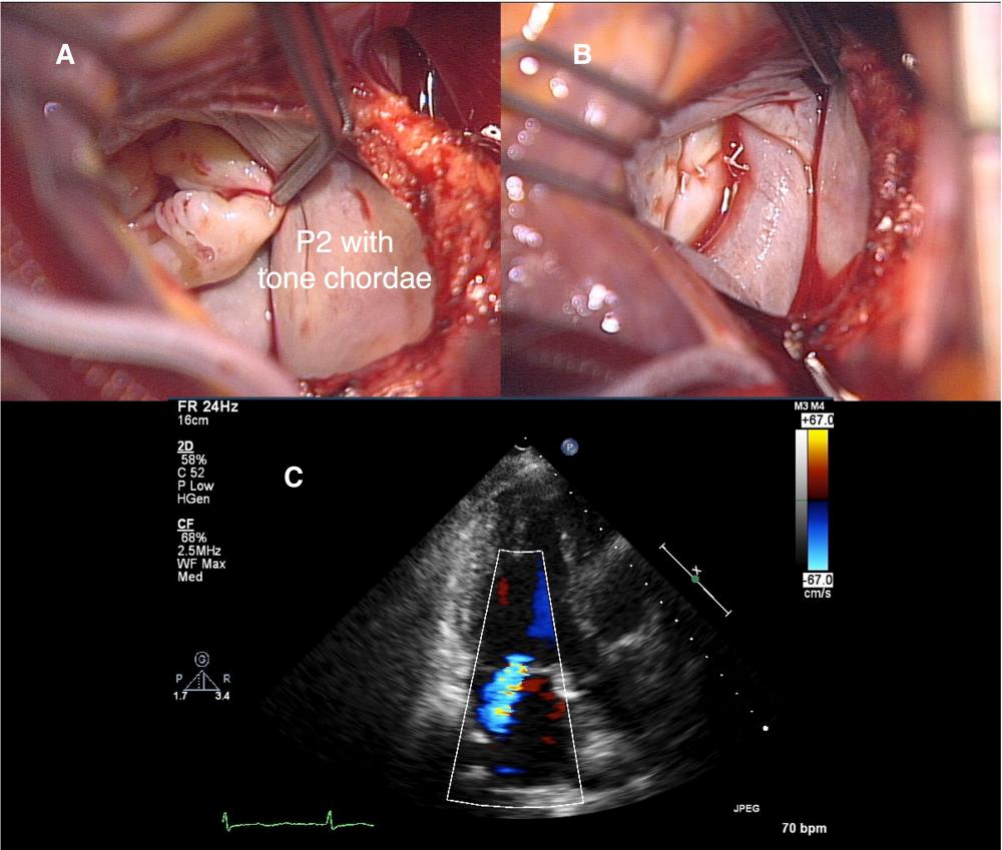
Initial surgical mitral valve repair. (*A*, *B*) Intraoperative images: P2 with ruptured chordae (*A*) and post-repair mitral valve (*B*). (*C*) Post-operative echocardiography: residual mild residual mitral regurgitation.
